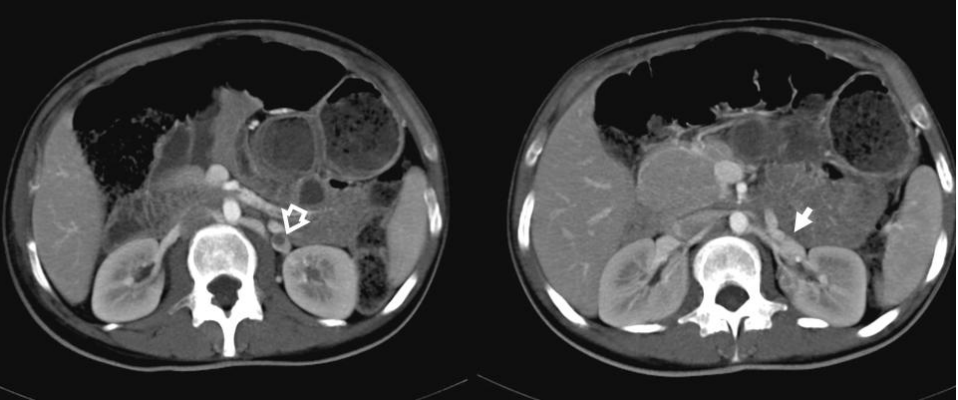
Contrast-enhanced CT-scan images with maximum intensity projections showing an intravascular filling defect in the left ovarian vein. 3D-reconstruction showing an intravascular filling defect in the left ovarian vein (open arrow) related to thrombosis. No thrombus progression into the left renal vein was observed (arrow).
